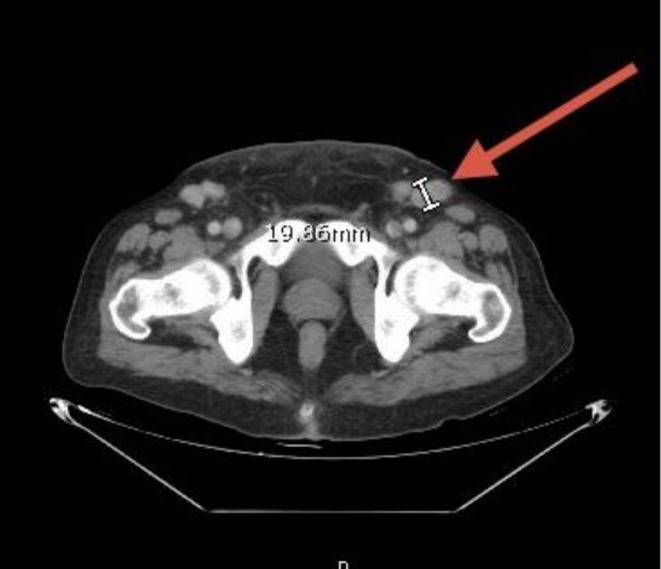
Inguinal lymphadenopathy. Contrast‐enhanced CT of the inguinal regions showing bilateral enlarged lymph nodes, supporting widespread lymphadenopathy.
